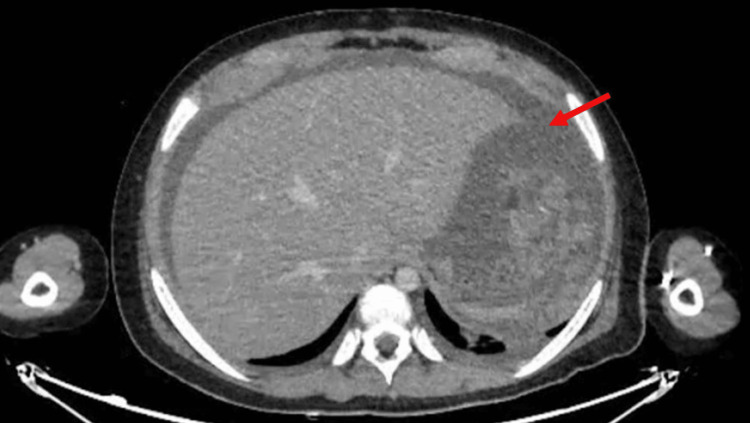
Abdominal Computed Tomography Red arrow indicating ascitic fluid
